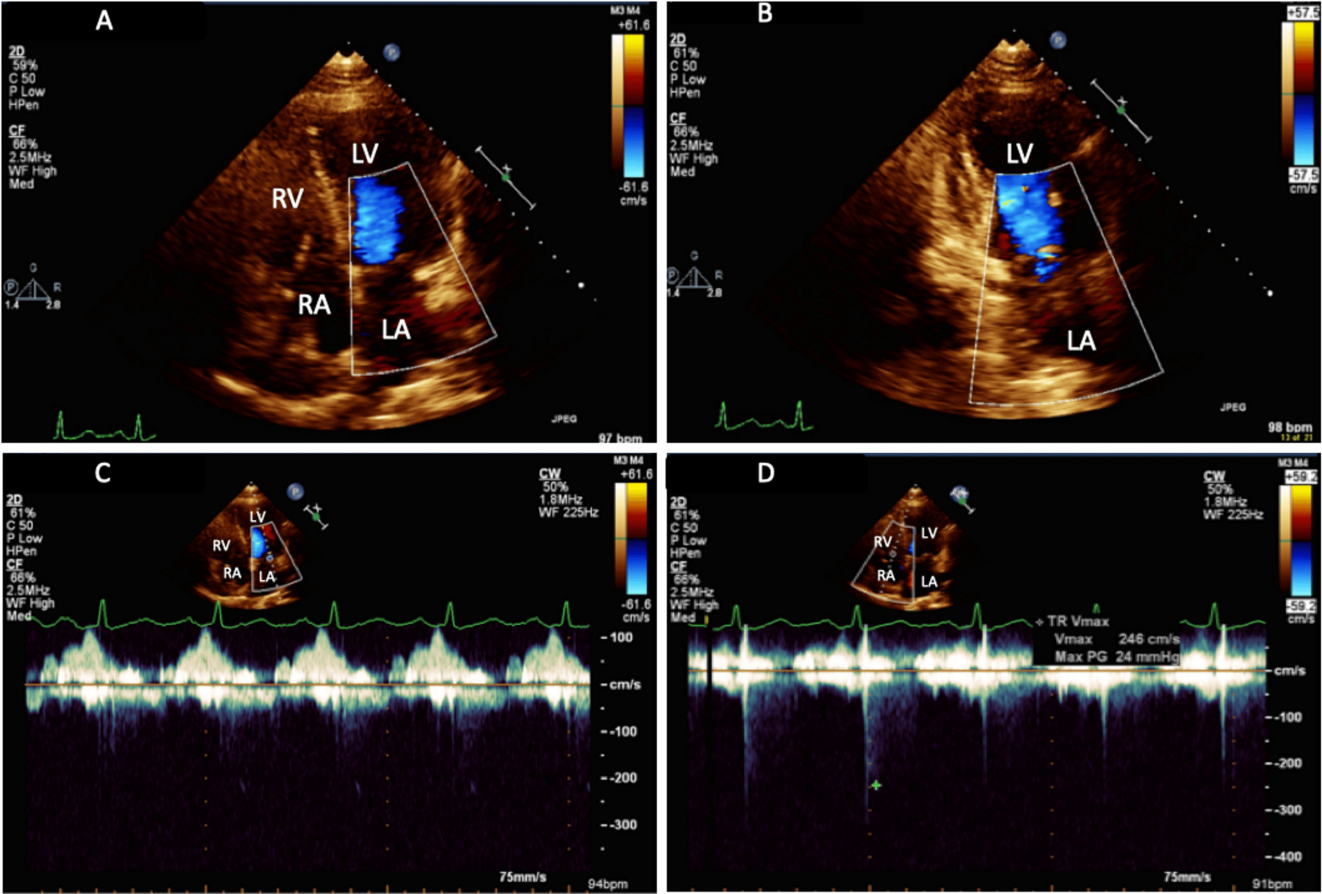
(A and B) TTE apical four-chamber view and two-chamber view with color Doppler, respectively, showing improved MR with reduced MR jet; (C) Apical four-chamber view showing trace MR by continuous wave Doppler in normal sinus rhythm; (D) Apical four-chamber view with color Doppler showing trace TR with TR Vmax 246 cm/s LA: left atrium; LV: left ventricle; RA: right atrium; RV: right ventricle; TR: tricuspid regurgitation; Vmax: peak velocity; TTE: transthoracic echocardiography; MR: mitral regurgitation
